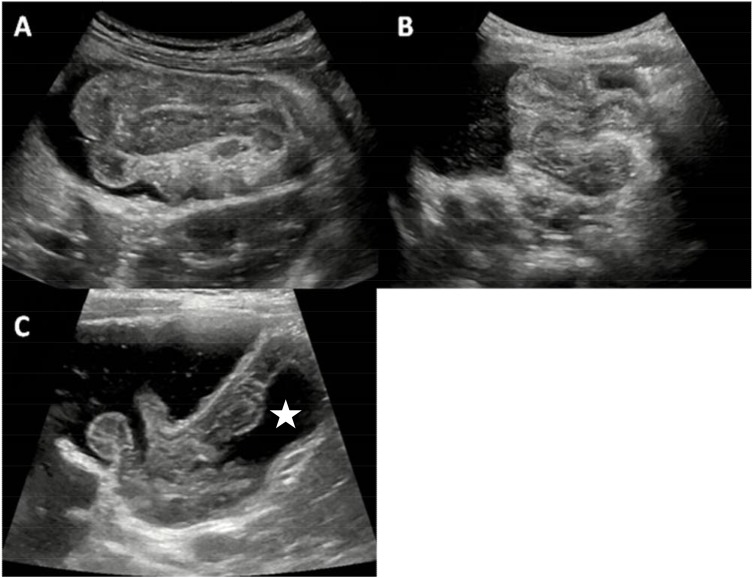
The ultrasonic manifestations during the ultrasound-guided reduction by saline enema. (A) Saline arrived at the intussuscipiens. (B) Intussusception gradually shortened. (C) Intussusception disappeared, saline passed through the ileocecal valve and fulfilled the terminal ileum (star).
